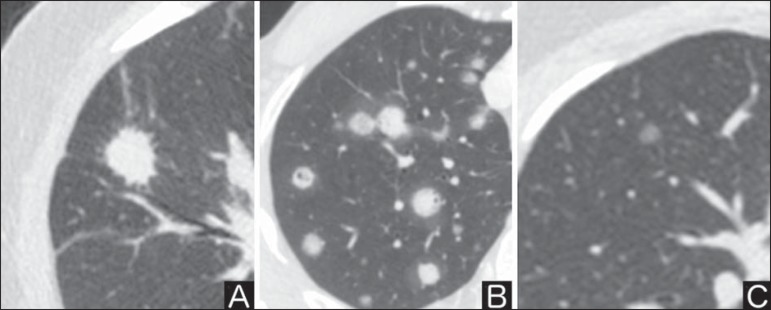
Attenuation.* Chest CT - lung window. A:
